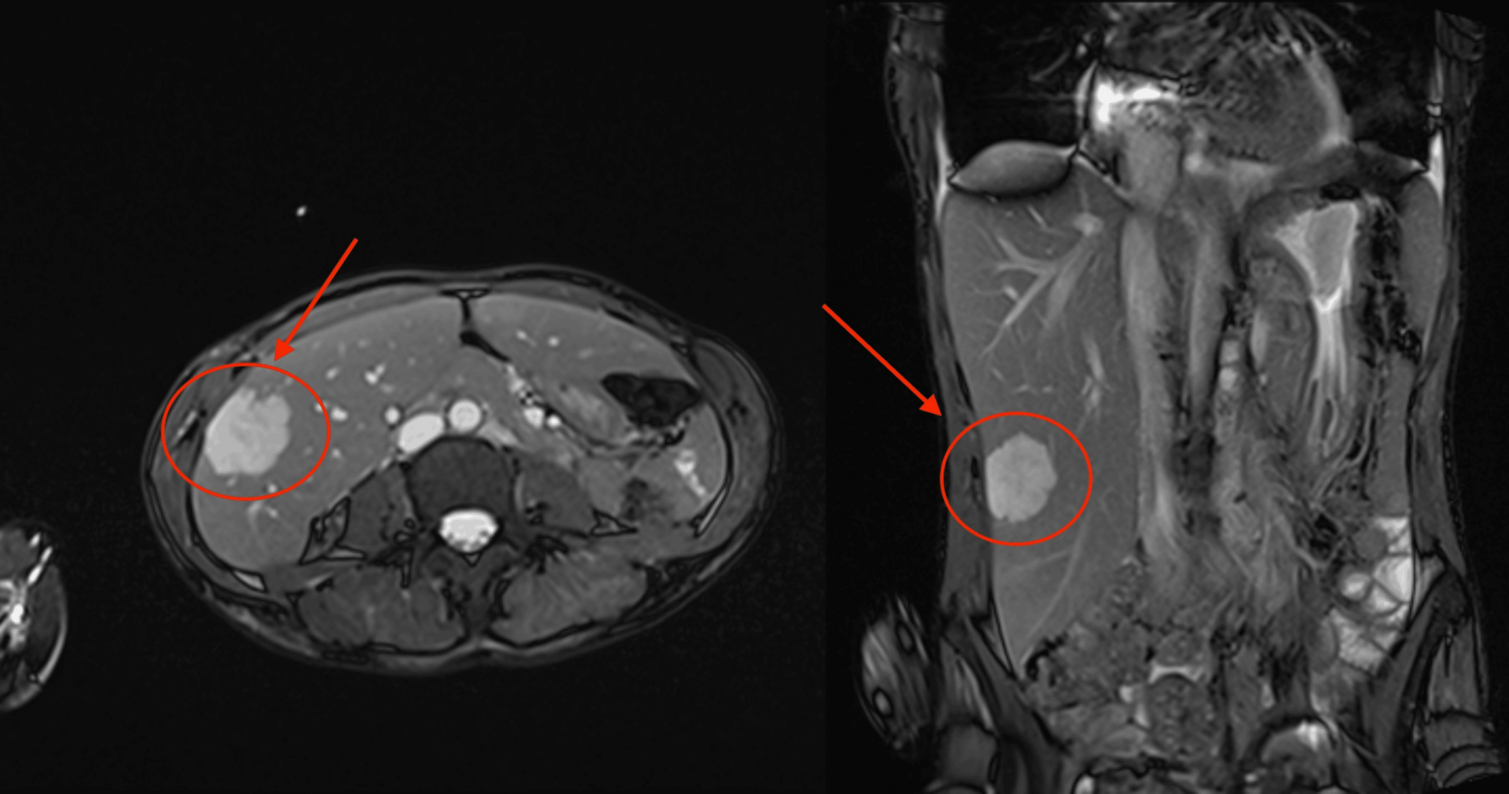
Liver MRI images further characterising the hepatic lesion as a benign haemangioma MRI: magnetic resonance imaging
